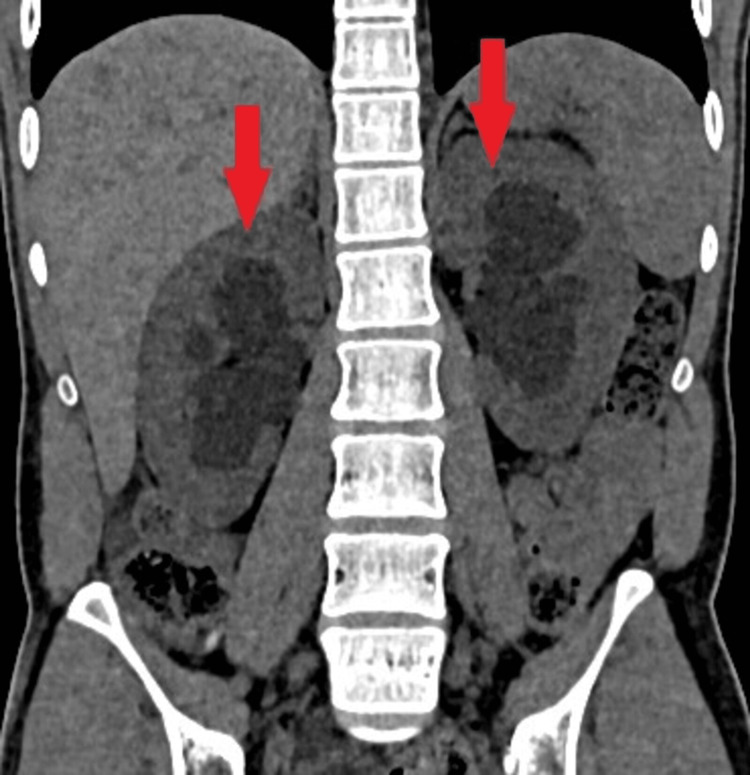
CT scan of the abdomen in a coronal section showing bilateral hydroureteronephrosis (two red arrows). CT: Computed tomography
